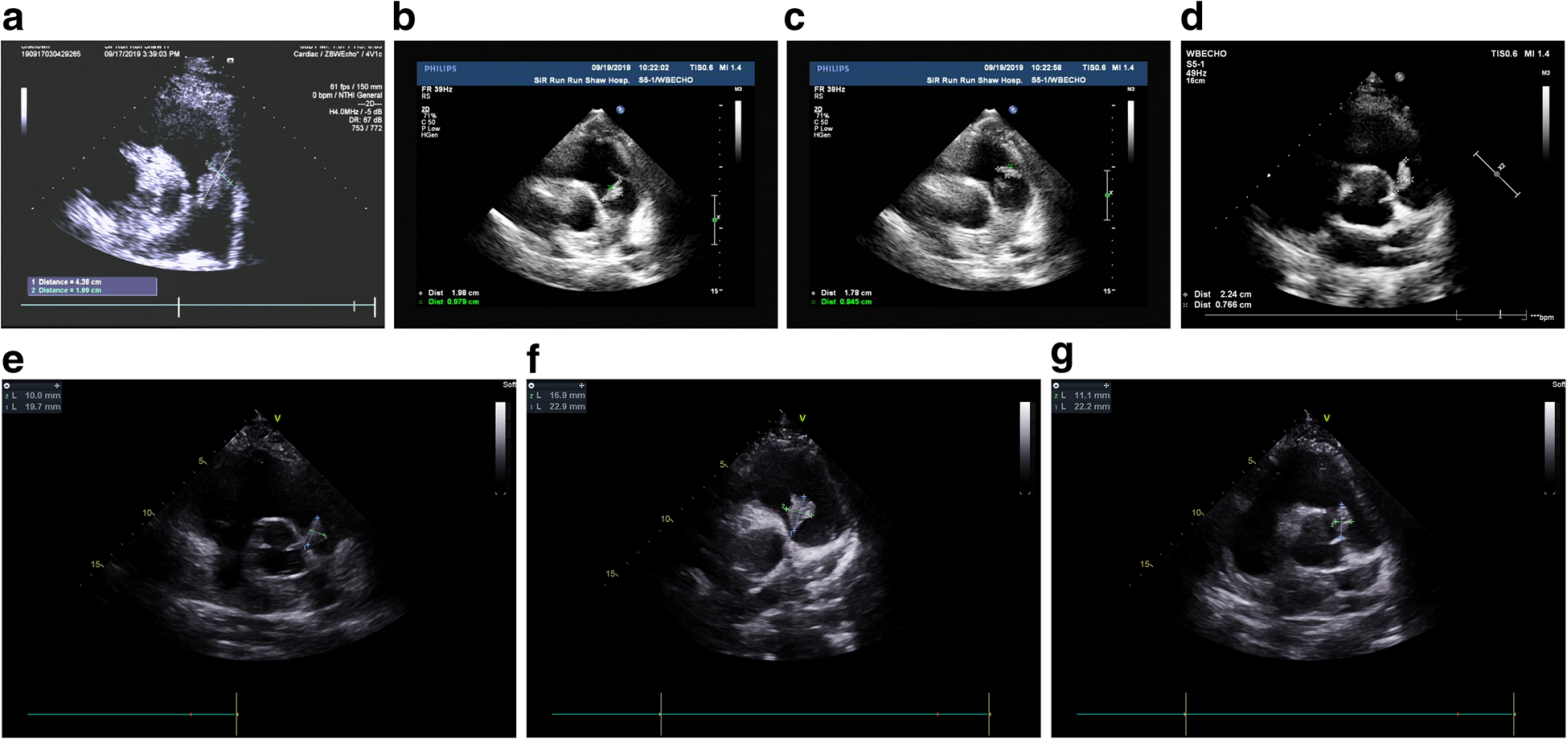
An ultrasonography series of ultrasonography showing PV vegetation from 1 day before admission to 2 days before the operation. Multiple cases of vegetations were observed, and one is measured in b-g. a was performed 1 day before admission, and b and c were performed on day 1 after admission. d, e, f and g were performed on days 8, 12, 16 and 26, respectively
